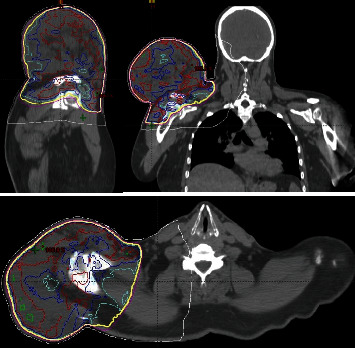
CT planning of tumour in sagittal, frontal, and transverse planes.
